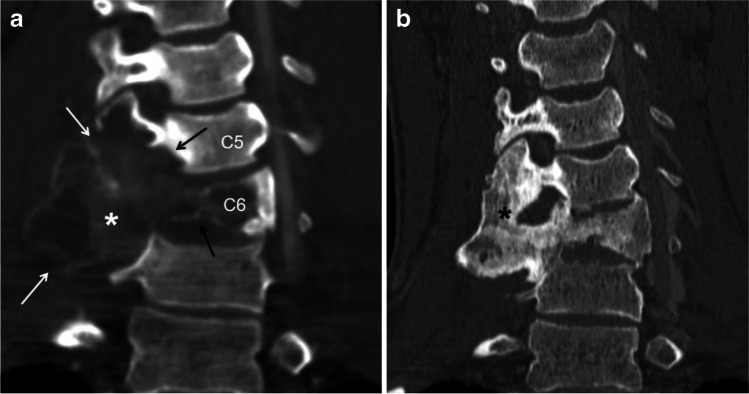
Aneurysmal bone cyst (ABC) of the cervical spine involving two consecutive vertebrae in a 16-year-old boy before and after percutaneous sclerotherapy with doxycycline. a A coronal reconstruction of a contrast-enhanced computed tomography (CT) scan image of the cervical spine using bone window shows a large, expansile lytic lesion (*asterisk*) involving the transverse processes (*white arrows*), articular pillars and body of C5 and C6 (*black arrows*). b A coronal reconstruction of a non-enhanced CT scan image of the cervical spine using bone window 6 years later after sclerotherapy shows complete interval healing of the lesion (*asterisk*) with diffuse sclerosis, remodeling of the bone and localized fusion at the site of the treated ABC
